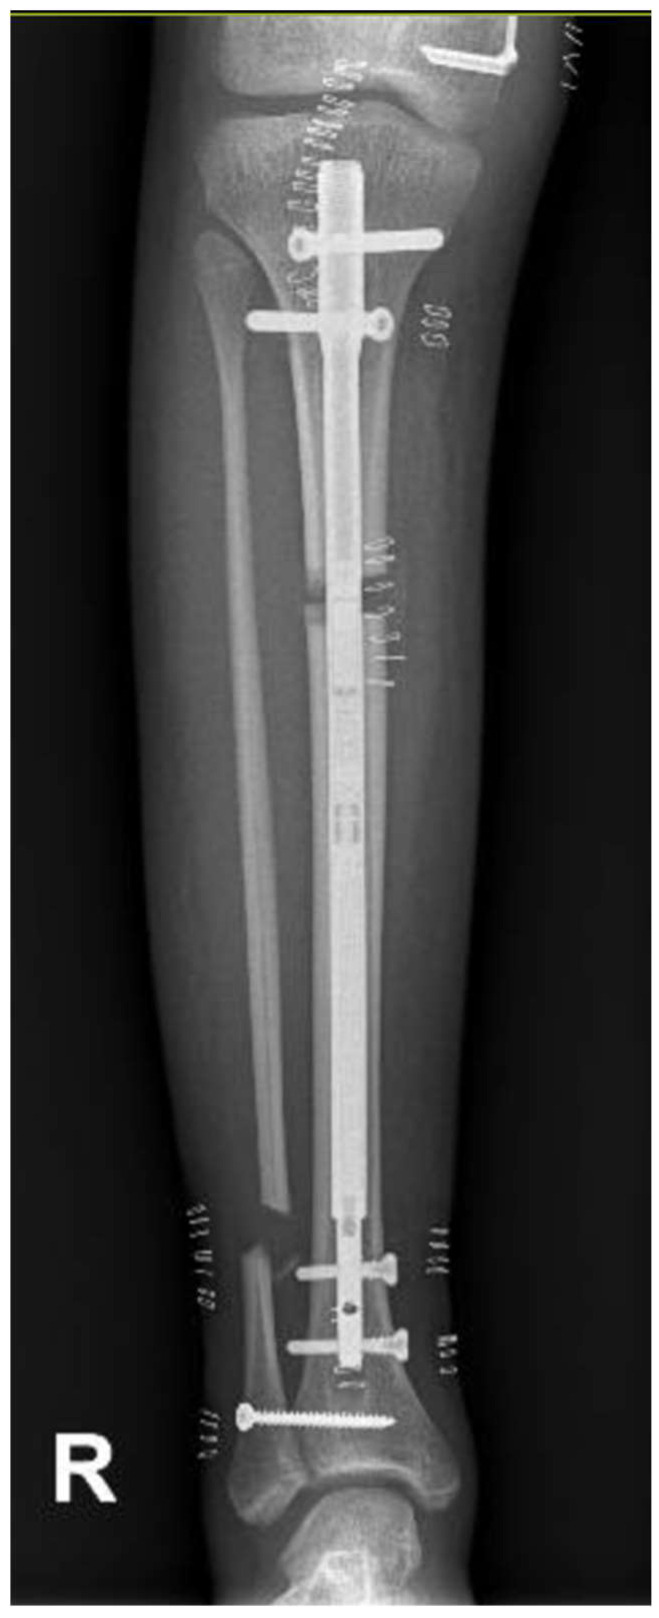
Radiographs showing proximal right tibial osteotomy and placement of an antegrade PRECICE^®^ expandable intramedullary nail and temporary femoral distal medial hemiepiphysiodesis.
